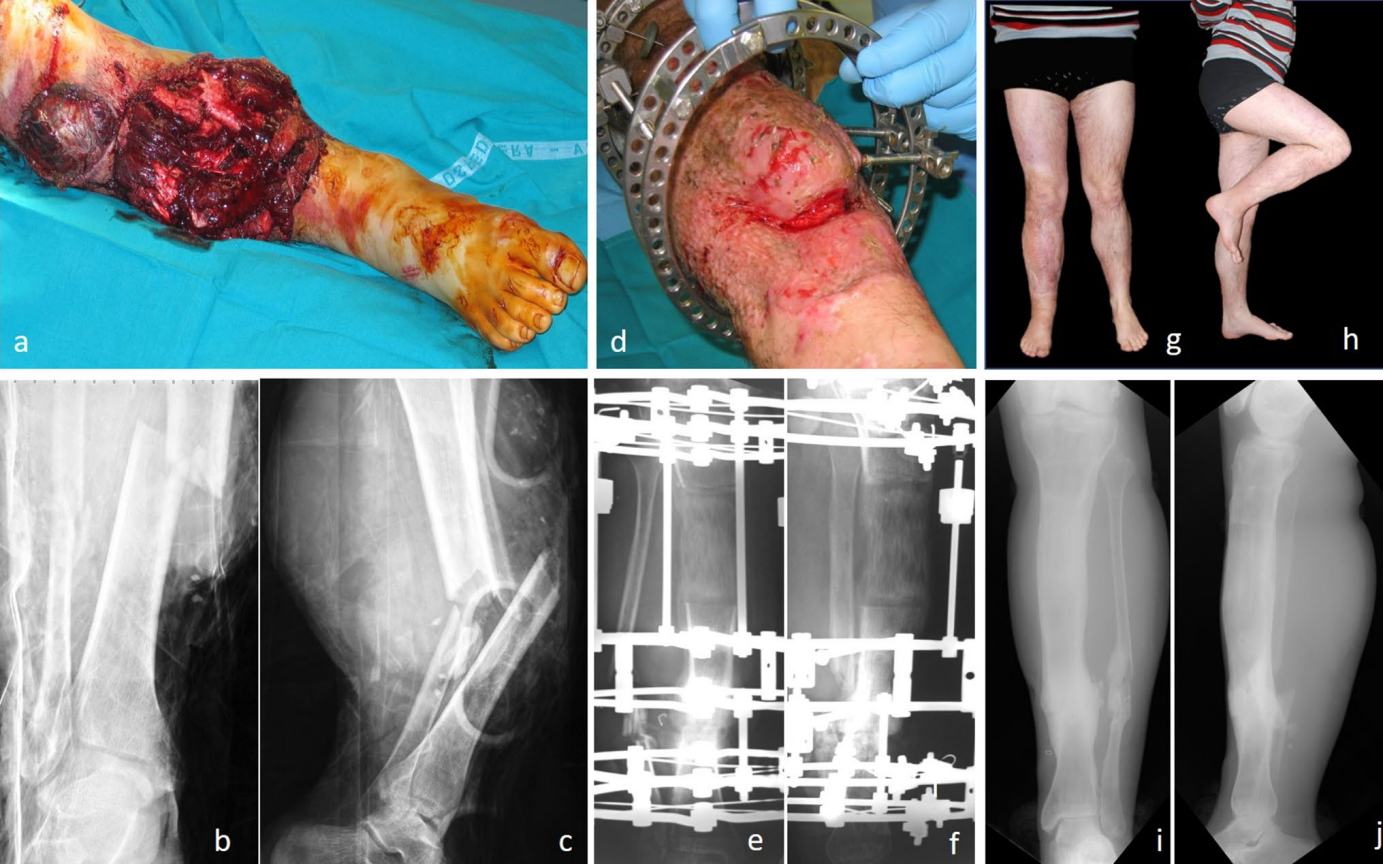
A 28-year-old male patient with an open fracture of the right tibia, Gustilo Anderson grade III-C with important bone and soft tissue defect: a right leg clinical presentation in operating room at arrival and preoperative X-rays on AP (b) and LL (c) views. Ilizarov bone transport: clinical image (d) and X-rays on AP (e) and LL (f) during treatment. Bone transport completed with good regenerate consolidation and docking union: clinical (g, h) and radiographic (i, j) images at 21-year follow-up
